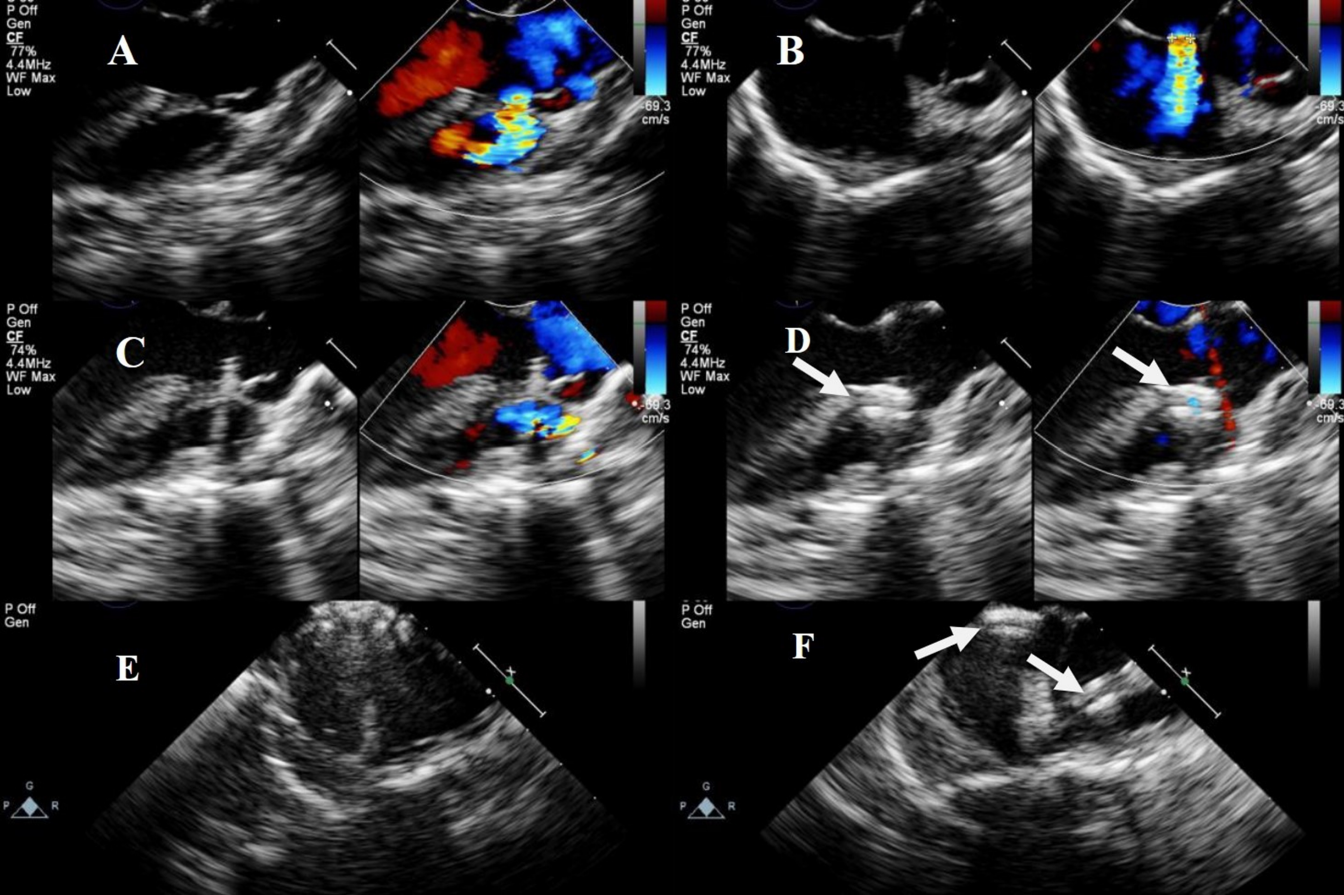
Transesophageal echocardiography of combined left
